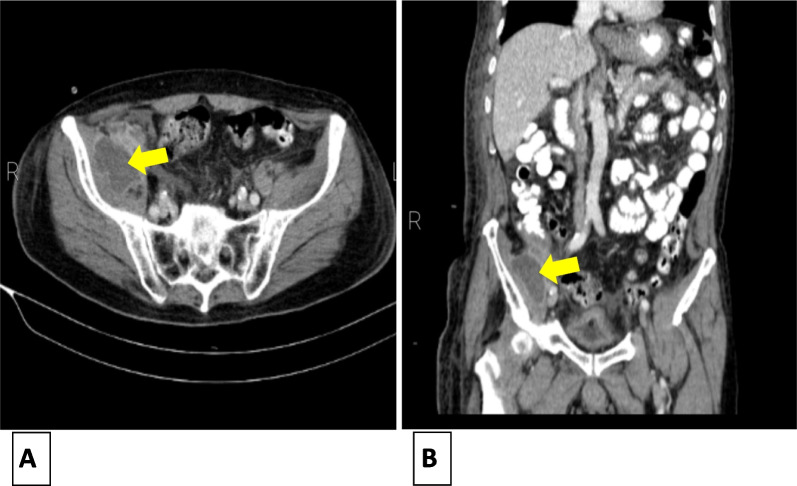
Abdominopelvic CT scan of the patient. Yellow arrows demonstrate collection in the right iliac muscle adjacent to the appendix. A Axial view. B Coronal view
